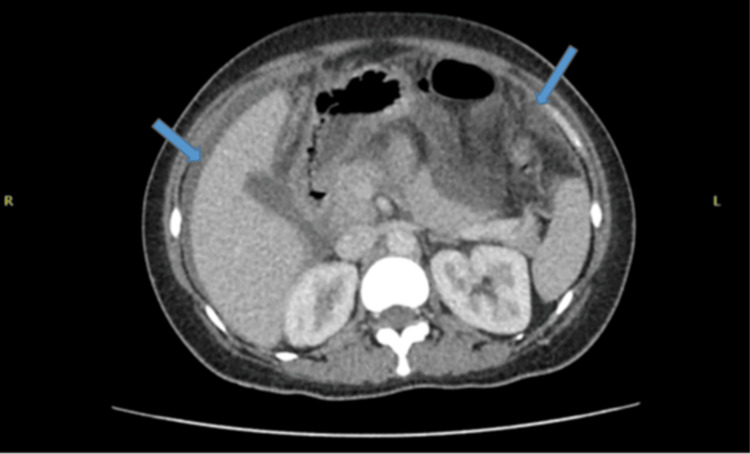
Cross-sectional CT images showing ascites and peritoneal thickening (blue arrows).
